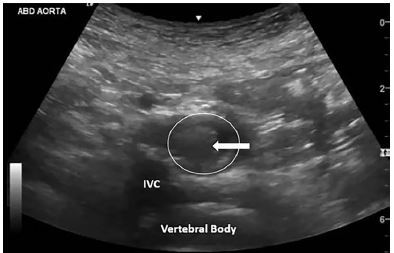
Point-of-care ultrasound transverse image of distal aorta (white circle) with hyperechoic intraluminal content (white arrow), representing thrombus.
